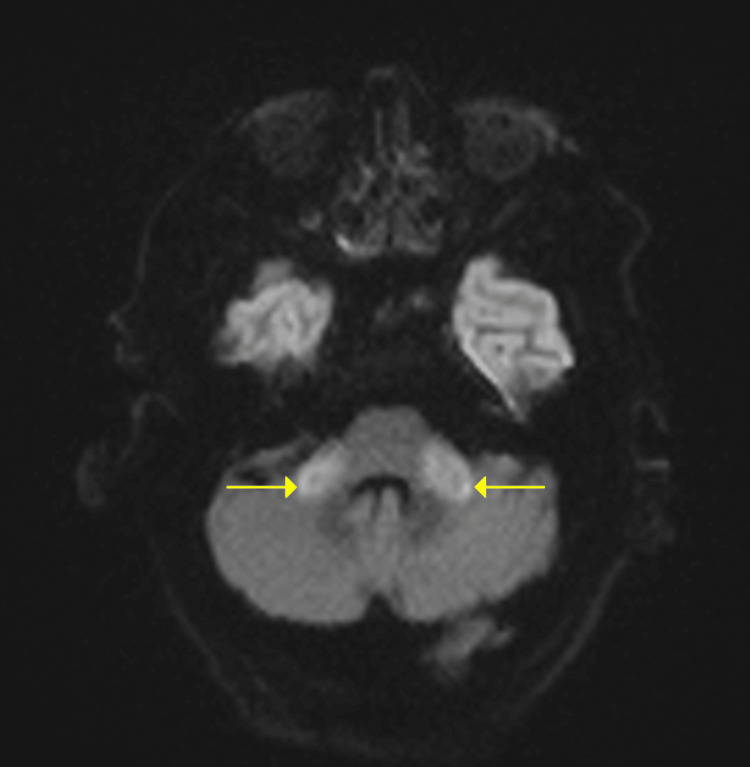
Axial DWI MRI demonstrating bilateral hyperintensity of the middle cerebellar peduncles (arrows), consistent with diffusion restriction in severe hypoglycemic encephalopathy. MRI: magnetic resonance imaging; DWI: diffusion-weighted imaging
